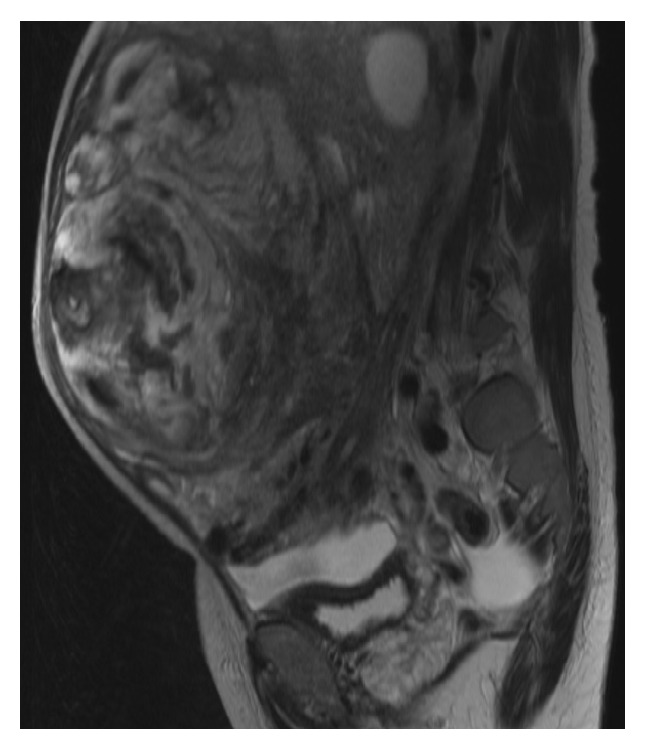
MRI image of a T2-weighted sagittal section. A huge abdominal tumor derived from the uterus, presenting with uneven intratumor signal.
